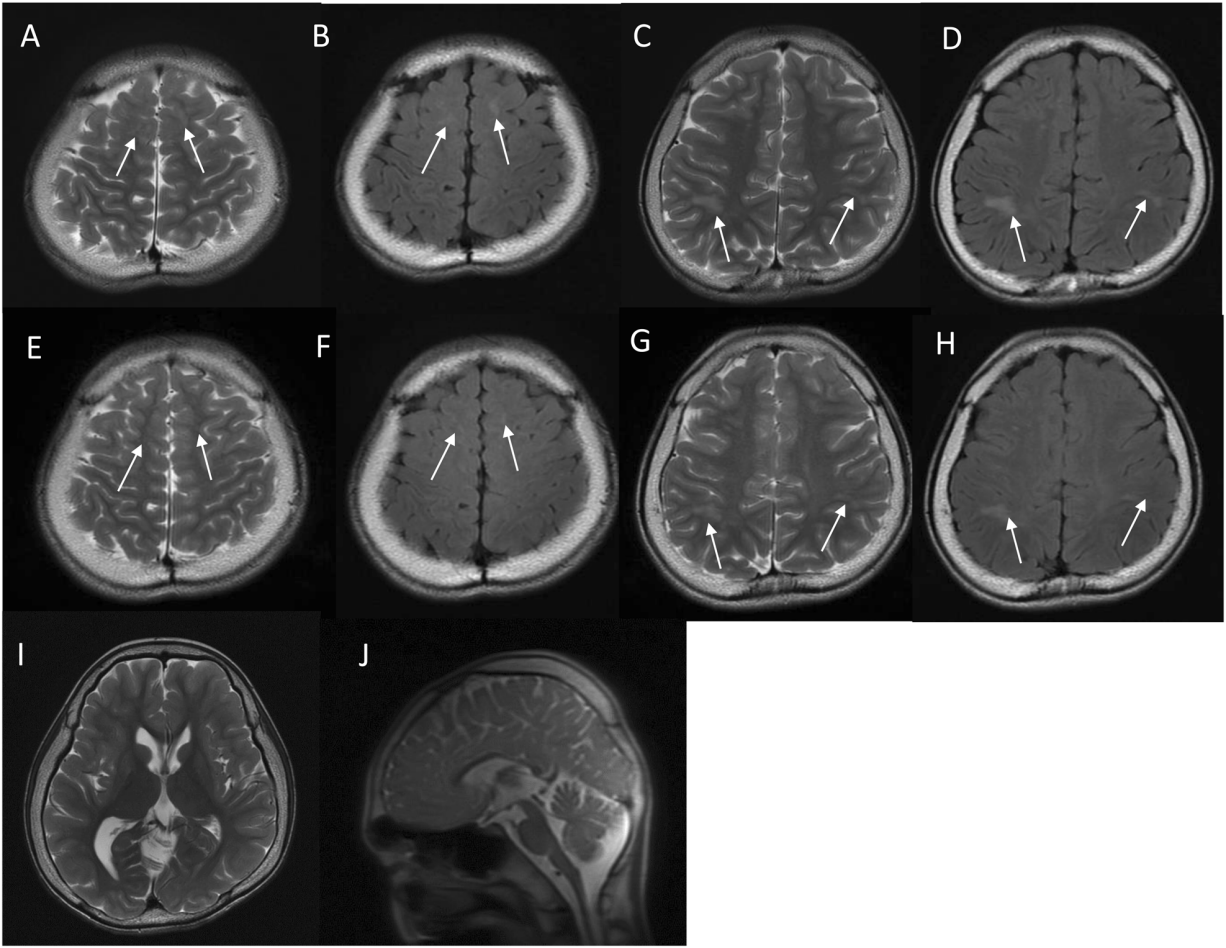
Brain magnetic resonance imaging (MRI) findings. Neuroimaging of the patient at A–D and I, J 11 years of age and E–H 15 years of age. A, C, E, G, I and J T2-weighted magnetic resonance imaging. B, D, F, and H Axial fluid-attenuated inversion recovery (FLAIR) magnetic resonance imaging. A–D Axial T2/FLAIR images showing hyperintense signals in white matter within the frontal and parietal lobes (arrows). The U-fibers were spared. I Axial T2-weighted imaging showed a slight enlargement of the left ventricle. J Sagittal T2-weighted imaging showed no morphological abnormalities in the corpus callosum or brainstem. E–H As in the image taken at 11 years of age, T2/FLAIR imaging showed hyperintense signals in white matter within the frontal and parietal lobes (arrows). The signal intensity of each lesion was lower in this image than in the one taken at 11 years of age.
